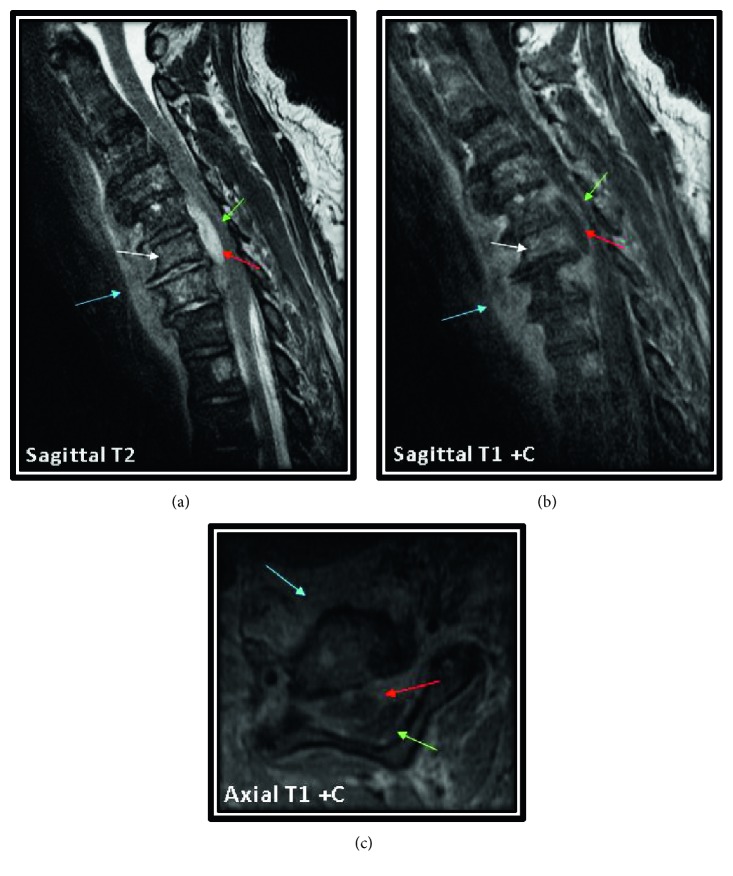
Sagittal and axial MRI scan of the cervical spine showing epidural abscess along the anterior margin of the cervical spinal cord (red arrow) causing cord compression from C5 through C7 (green arrow). There is also anterior subligamentous abscess from C4 through T2 (blue arrow) and marrow edema and enhancement involving C6 and C7 vertebral bodies (white arrow) with increased signal in the intervertebral disc space at C6-C7 consistent with osteomyelitis and discitis.
